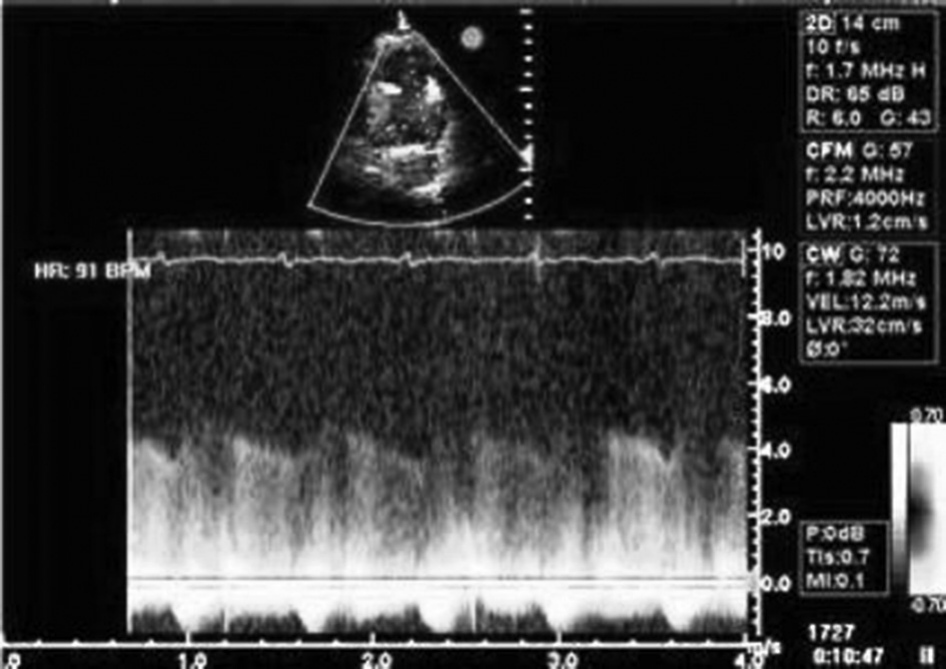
Parasternal short axis view shows high velocity continuous flow of patent ductus arteriosus
